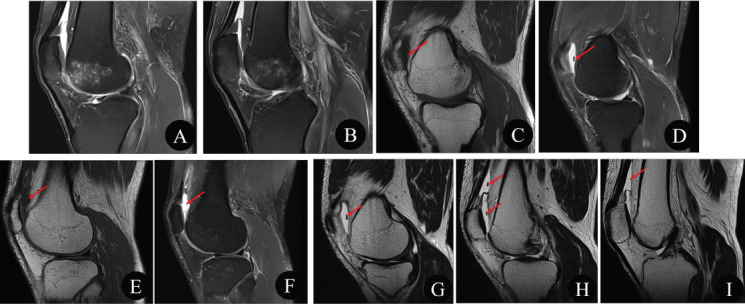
Three days after the second presentation, the third presentation of the right knee MRI examination. Sagittal PDWI-fs (A-B) showed that the area of subchondral bone marrow edema increased compared to three days ago, and the cartilage fracture and joint capsule effusion were basically the same. Sagittal T1WI (C and E) and PDWI-fs (D and F) showed two loose bodies (red arrows). The sagittal PDWI sequence of 2mm slice thickness without fat suppression (G-I) showed four loose bodies in the joint capsule (red arrows).
